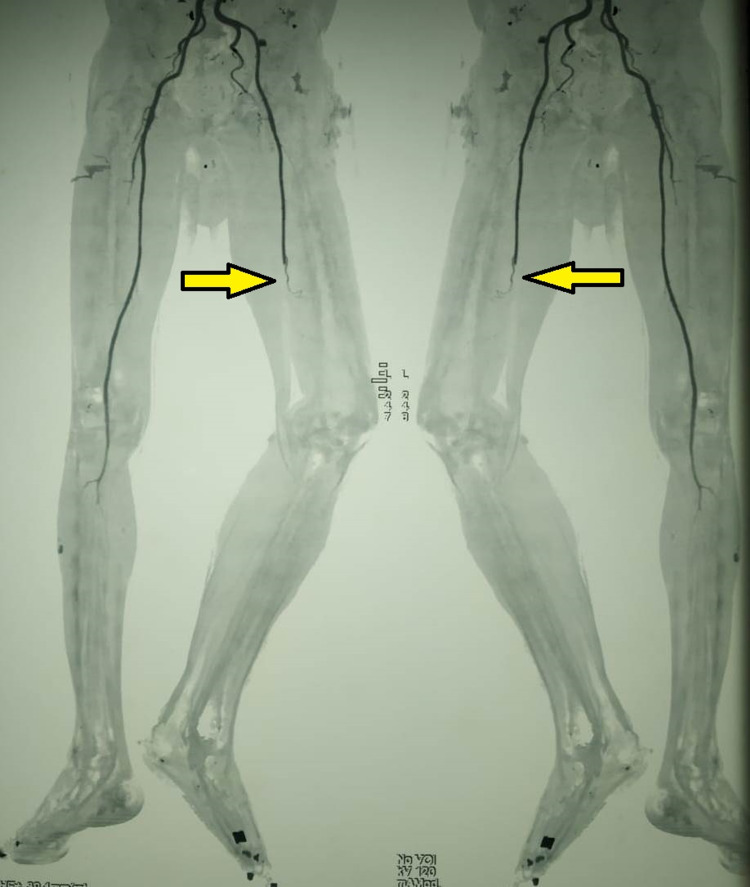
CT subtraction angiogram showing complete occlusion of the mid superficial femoral artery, popliteal artery, and infra popliteal artery with poor collateral status (Arrow)
